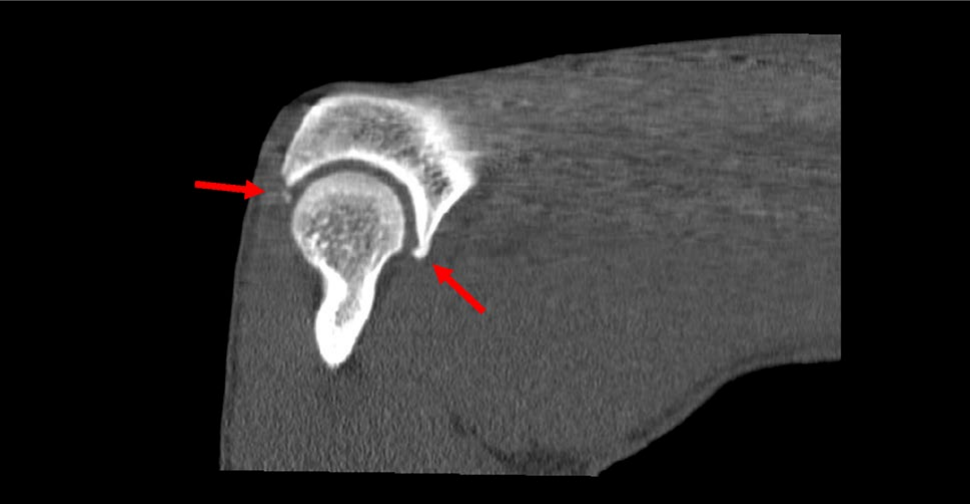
CT scan of the right elbow of patient no. 2 (boxer) displaying osteophytes at the tip of the olecranon as well as the coronoid process (sagittal plane)
